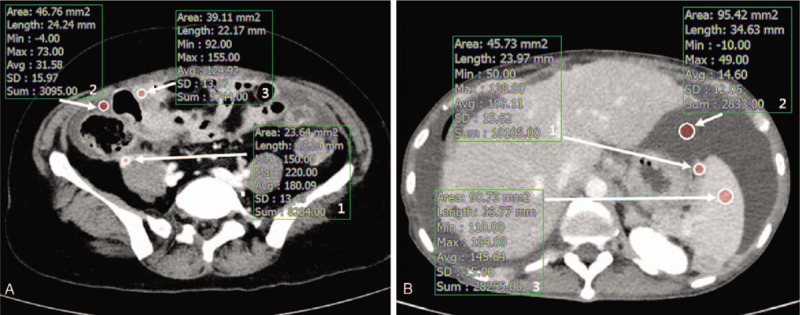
Obtaining ROIs for quantitative image analysis. The average CT attenuation and standard deviation (SD) of the peritoneal implant and were obtained from a single ROI drawn in the most enhancing solid portion of the lesion (Box 1). The adjacent ascites were measured for reference tissue (Box 2). (A) The virtual monoenergetic image at 40 keV in 52-year-old ovarian cancer patient. For cases in which the peritoneal implant was located near the bowel, mean attenuation and SD of the adjacent small or large bowel wall was additionally obtained (Box 3). (B) The virtual monoenergetic image at 40 keV in 62-year-old ovarian cancer patient. For cases in which the peritoneal implant was located in the surface of liver or spleen, the mean attenuation and SD of the adjacent liver or splenic parenchyma was additionally obtained (Box 3).
